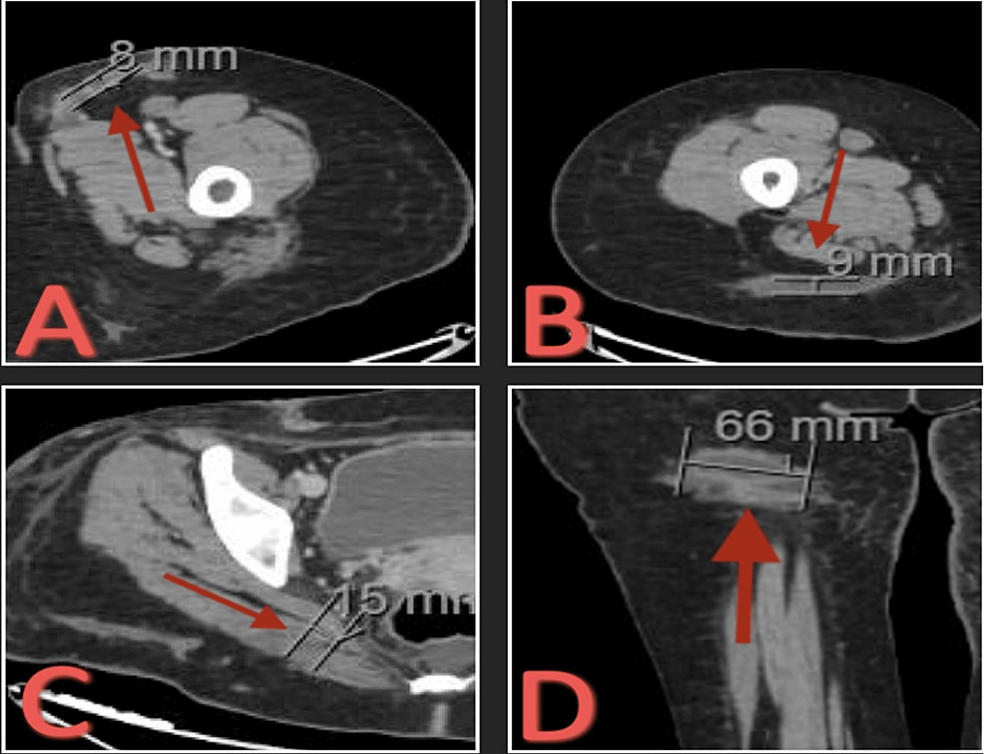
CT bilateral lower extremities with contrast. (A) Transverse view of 8 mm small-sized remnant sub-centimeter collection in the left thigh. (B) Transverse view of 9 mm small-sized remnant sub-centimeter collection in the right thigh. (C) Transverse view of 15 mm small-sized remnant sub-centimeter collection in the right glute. (D) Coronal view of 66 mm small-sized remnant sub-centimeter collection in the right thigh.
